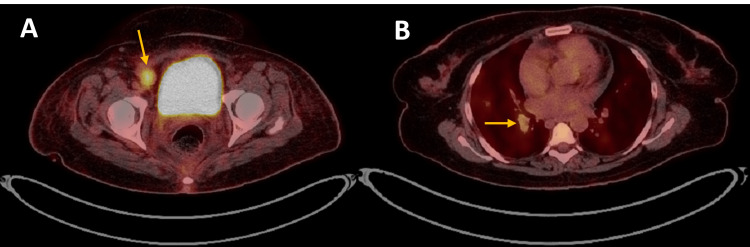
Axial 18F-FDG PET/CT (Chest and pelvis sections) There is (A) FDG avid right common iliac and right external iliac lymphadenopathy (orange arrow in A) that measures 2.3 cm^2^ (SUVmax 6.3), and (B) small nodular opacities in the right middle lobe. The largest lung nodule (orange arrow in B) measures 1.4 cm^2^ (SUVmax 1.8). 18F-FDG PET/CT: Flourine-18 fluorodeoxyglucose positron emission tomography/computed tomography
